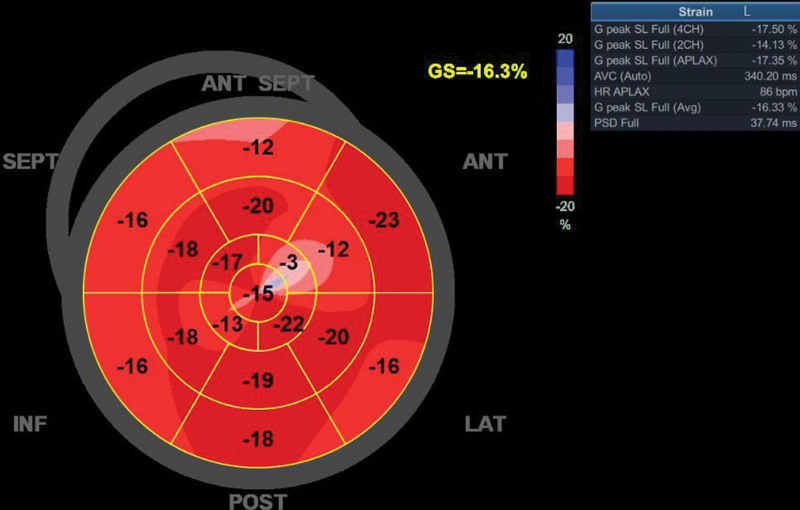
The echocardiography when the child was 10 revealed reduced LV-GLS. GLS = global longitudinal strain, LV = left ventricular.
